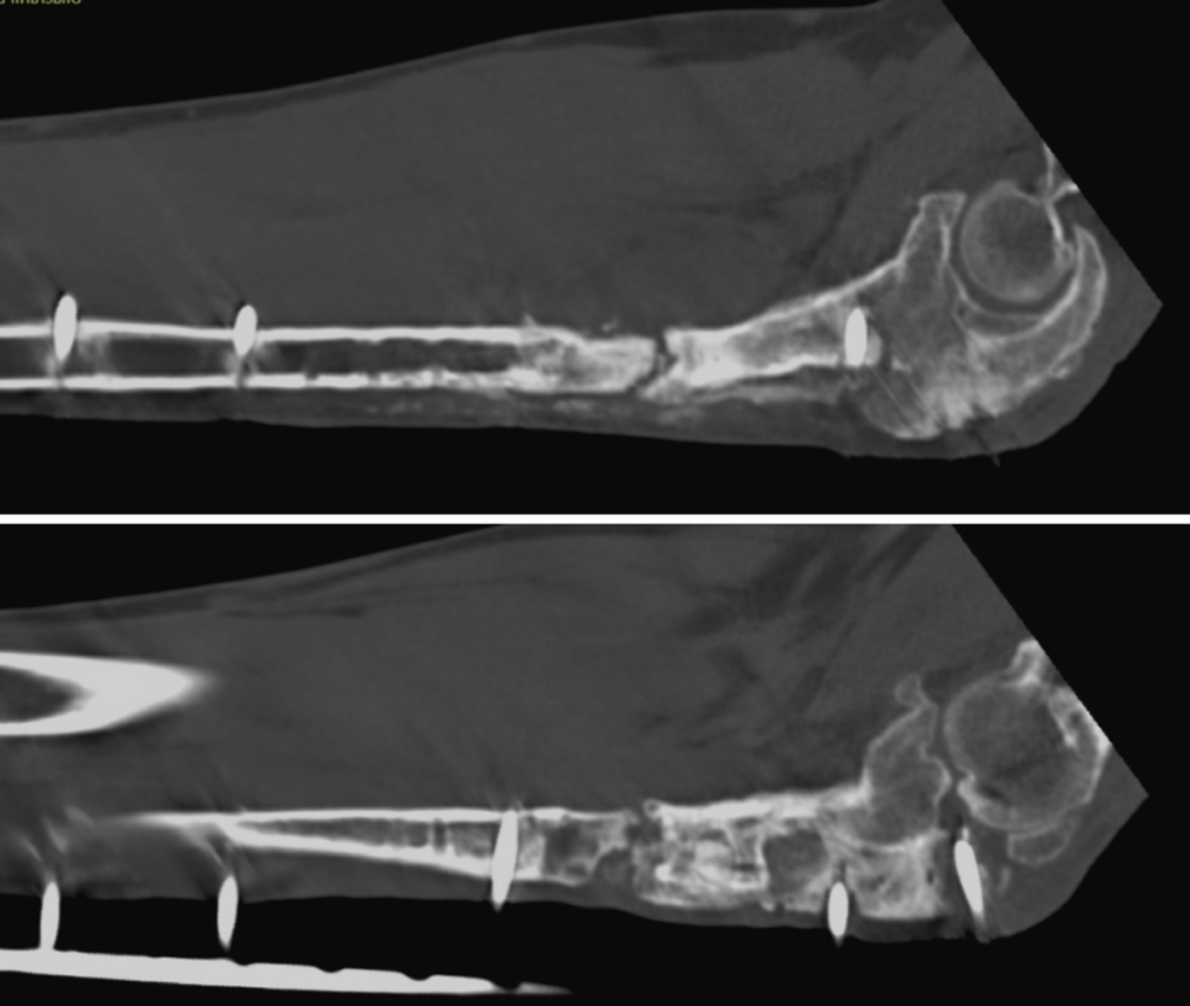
CT scan after six months The distal part of the graft is united while the proximal part is not.
